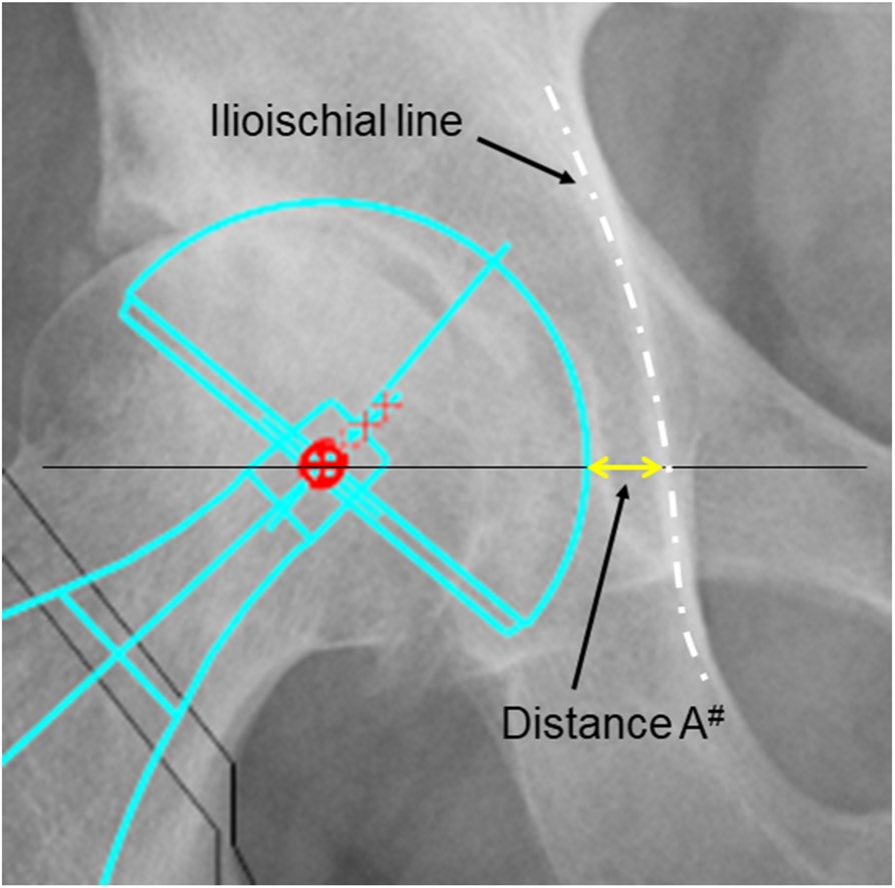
Two-dimensional templating. The image shows the usage of the ilioischial line for determining the target offset position of the acetabular cup. The exact distance from the ilioischial line to the acetabular cup is unclear on CT axial images. CT, computed tomography
